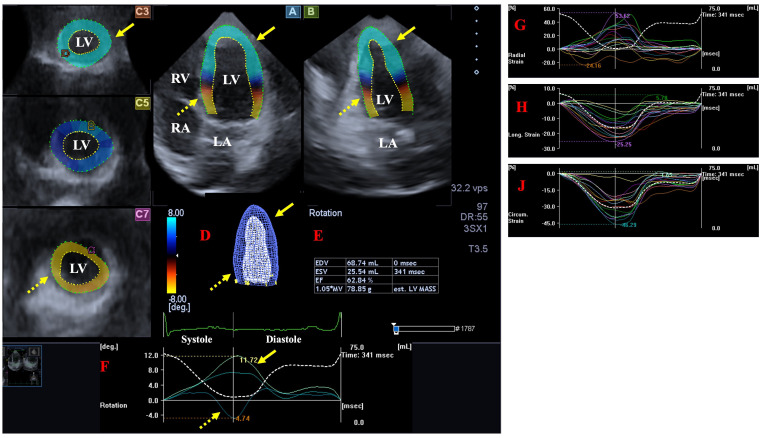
Three-dimensional (3D) speckle-tracking echocardiographic examination of the left ventricle (LV) in a healthy subject: apical longitudinal four-chamber (A) and two-chamber views (B) and apical (C3), midventricular (C5) and basal (C7) short-axis views are demonstrated together with 3D cast of the LV (D), volumetric LV parameters and LV ejection fraction (E) and time-LV volume changes (dashed white line). Time—apical (yellow arrow) and basal (dashed yellow arrow) LV rotations (F) and global (white line) and segmental (coloured lines) time—radial (G), longitudinal (H) and circumferential (J) LV strain curves are also shown. Abbreviations. LV = left ventricle, LA = left atrium, RA = right atrium, RV = right ventricle.
